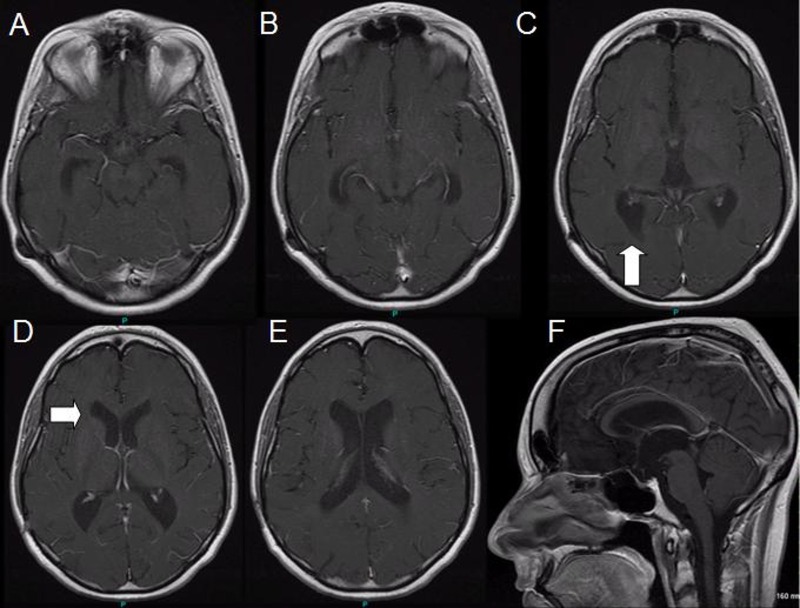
Post-contrast axial and sagittal MRI sequences one year after diagnosis and initiation of radiation therapy. There is resolution of tumor burden with mild residual ventriculomegaly.
